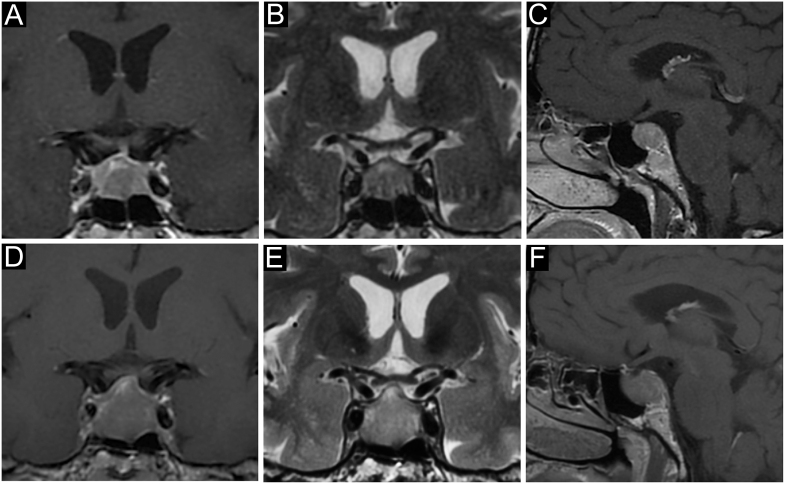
A case of non-functioning gonadotroph PitNET in a 65-year-old man. This patent was referred because of an incidental sellar mass on cerebral MRI for unexplained episodes of brief unconsciousness, in the setting of recent hypertension. Pituitary MRI was consistent with a non-invasive PitNET with initial suprasellar extension (A, B, C)*. Clinical examination, endocrinological evaluation and visual field (VF) examination were unremarkable, and follow-up was advised. A slow worsening of pituitary function was noticed over the next 4 years, requiring L-thyroxine and then transdermal testosterone replacement. A slow progression towards the optic chiasm was noticed (D, E, F)*, and transsphenoidal surgery was planned despite normal VF. Noteworthily, minimal temporal quadrantopsia was noticed just before surgery. No recurrence was found at a 2-year follow-up, and transdermal testosterone was recently withdrawn upon urological advice because of a worsening benign prostate hypertrophy. *(A and D) coronal contrast-enhanced T1-weighted, (B and E) coronal T2-weighted and (C and F) sagittal contrast-enhanced T1-weighted.
